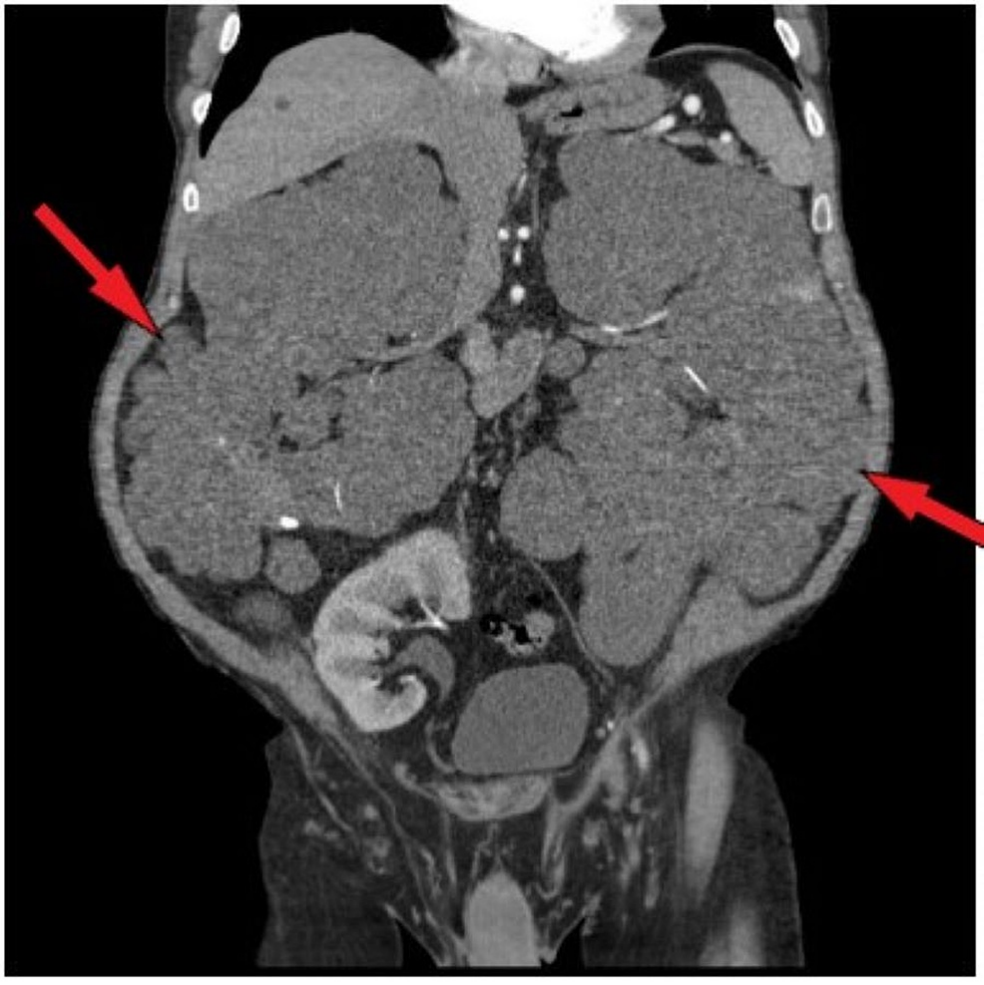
CT scan (2012), coronal view of enhancing cysts of the native kidneys. Left kidney: inner polar region enhancing mass measuring 4.5 cm (red arrow). Right kidney: inner polar region enhancing mass measuring 8.0 cm (red arrow). CT: computerized tomography
